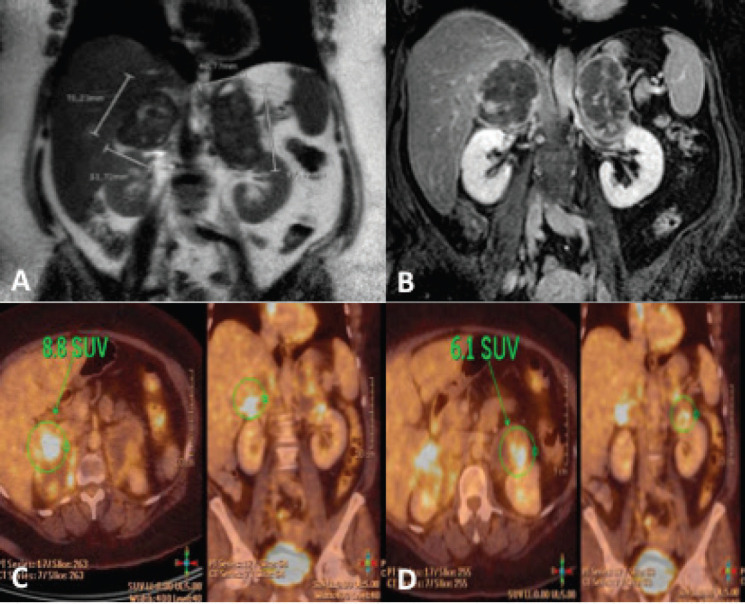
(a) Coronal section on MRI in T2-weighted sequence showing bilateral adrenal masses (right of 75 × 52 mm and left of 98 × 49 mm). (b) Similar section in T1-weighted with fat suppression and Gadolinium sequence. (c and d) PET-CT showing peripheral metabolic increase in nodular formations in right and left adrenal glands, with 8.8 and 6.1 SUV, respectively.
